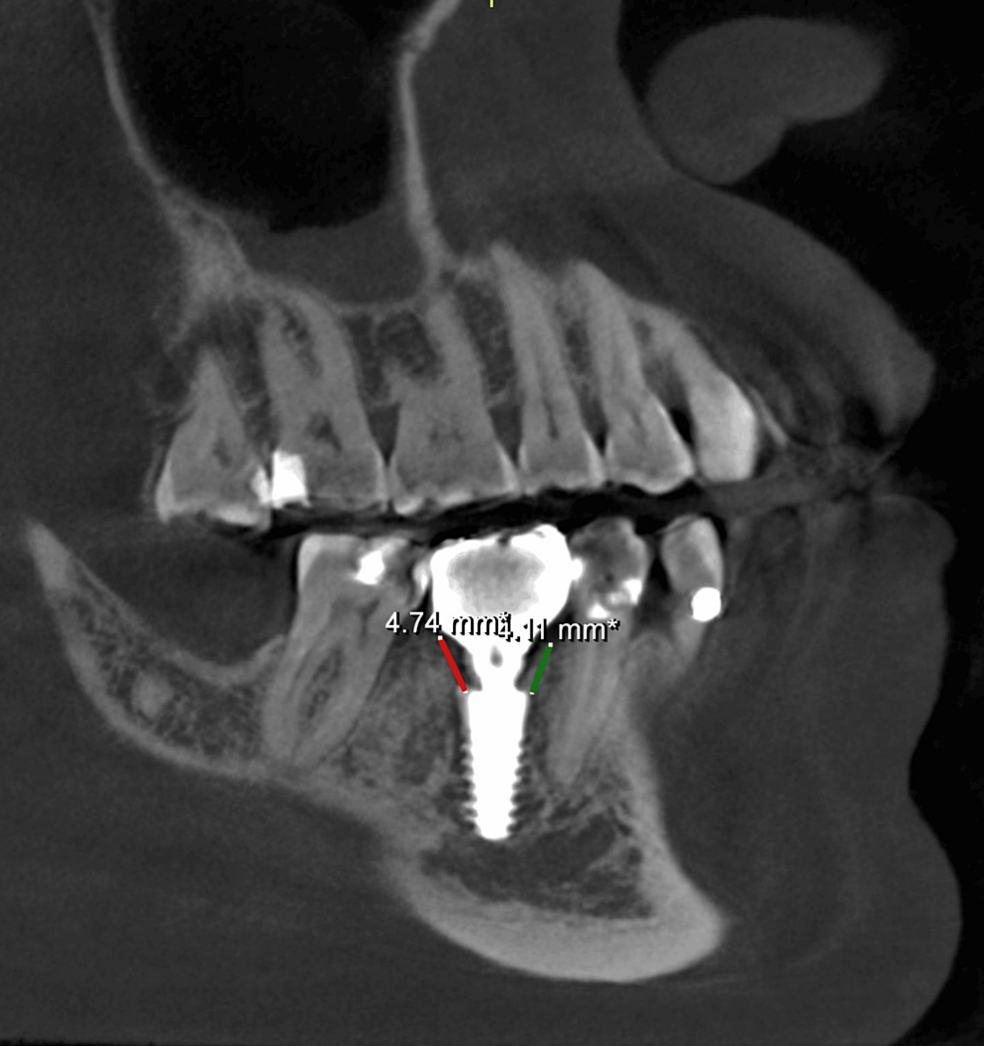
Cone beam CT scan showing the marginal bone-level measurements in mesial and distal sites.
